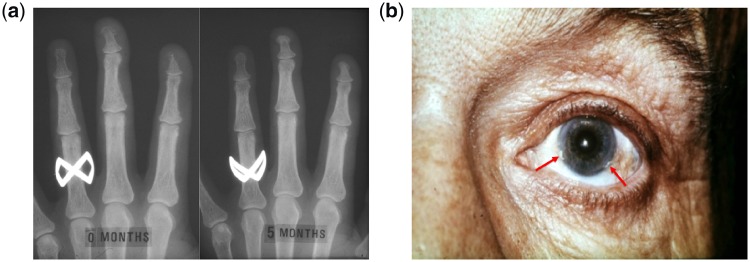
(a), Left Panel) Shows an X-ray of the left hand of a 27-year old female with ESRF taken immediately prior to her commencing activated vitamin D3 therapy. It demonstrates bone resorption of the terminal phalanges. (a, Right Panel) Shows the X-ray taken 5 months later and shows remarkable bone remodelling, secondary to activated vitamin D3 therapy, most evident at the terminal phalanx of the second finger. (b) Shows a photograph of the left eye of a 44-year old male with ESRF. It demonstrates calcification of the corneal limbus at the 5 and 8 o'clock positions.
